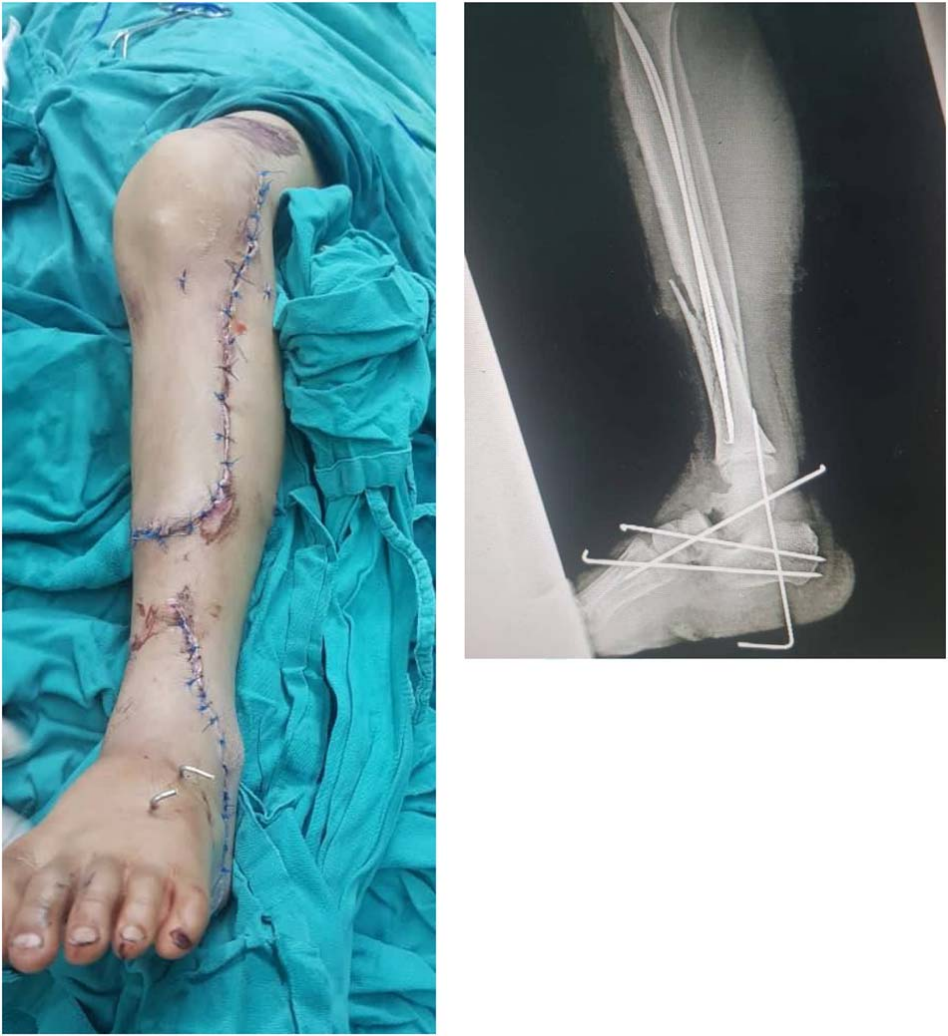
Displays the leg after suturing the skin flap, along with the X-ray image following the installation of the K-wires.
